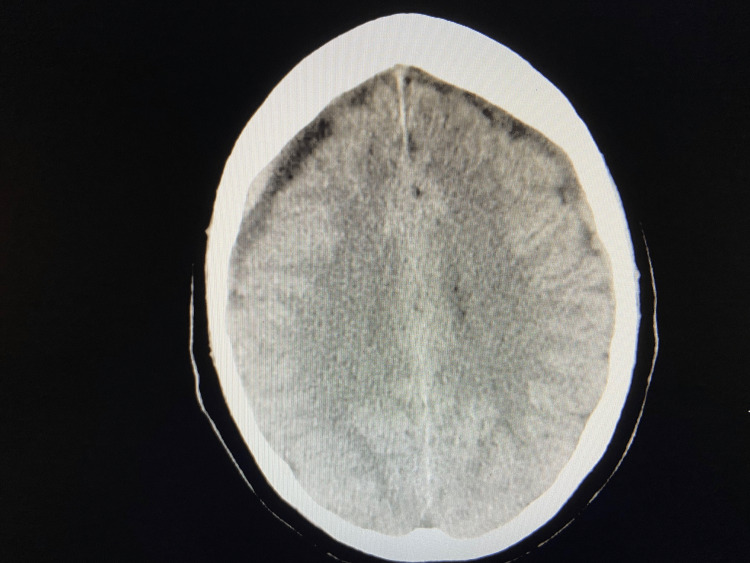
A CT of the brain (without contrast) showed bilateral subdural hematomas within the lateral convexities
